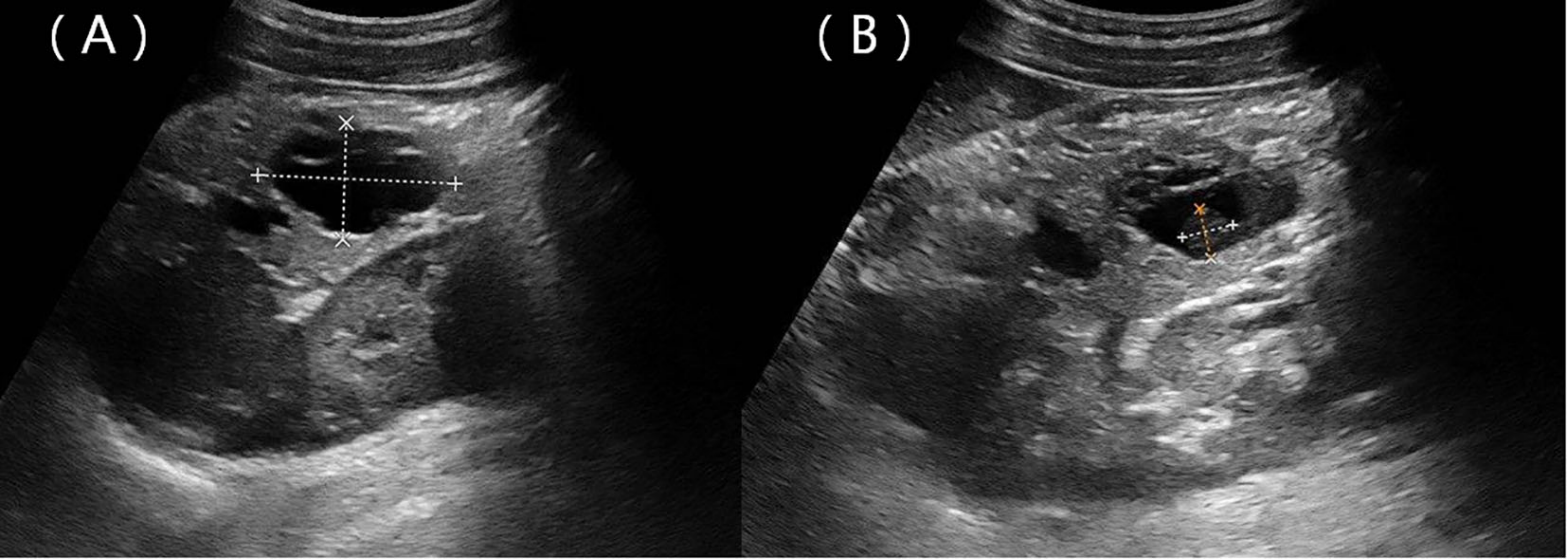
Ultrasound showing a hypoechoic mass located in the body and tail of the pancreas with clear margins (A) and a nodule with strong echoes at the edge (B).
